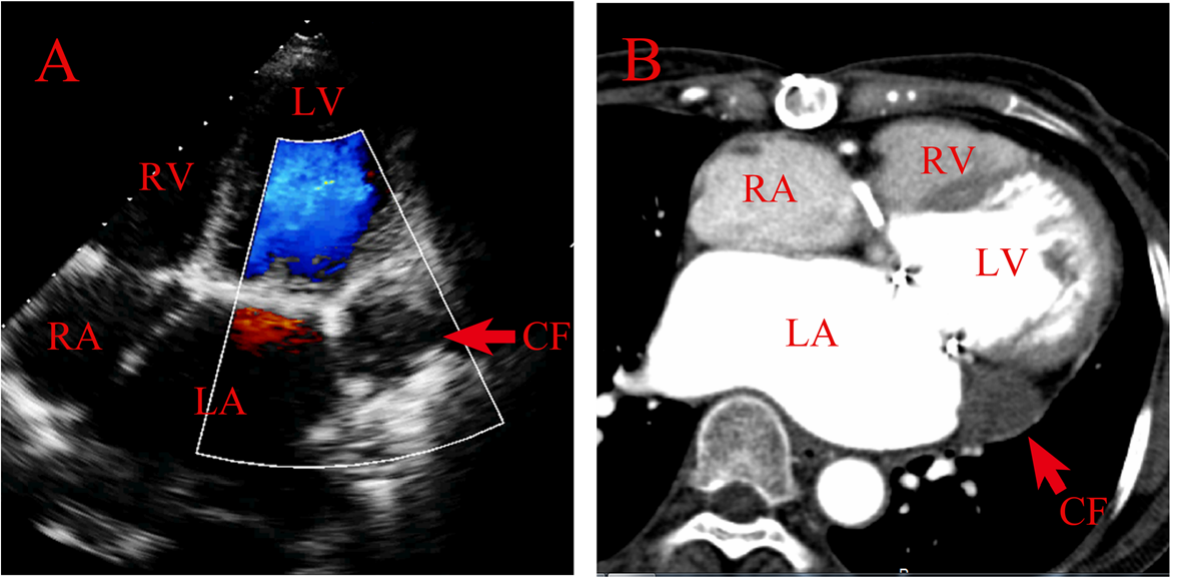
a Follow-up transthoracic echocardiogram in apical four-chamber view showed no blood communication between the clot-filled pseudoaneurysm and the left ventricle. The psedoanurysmal sac was filled with clot. b Follow-up enhanced computed tomography revealed no enhancement of the pseudoaneurysm. RV: right ventricle. RA: right atrium. LV: left ventricle. LA: left atrium. CF: clot formation
